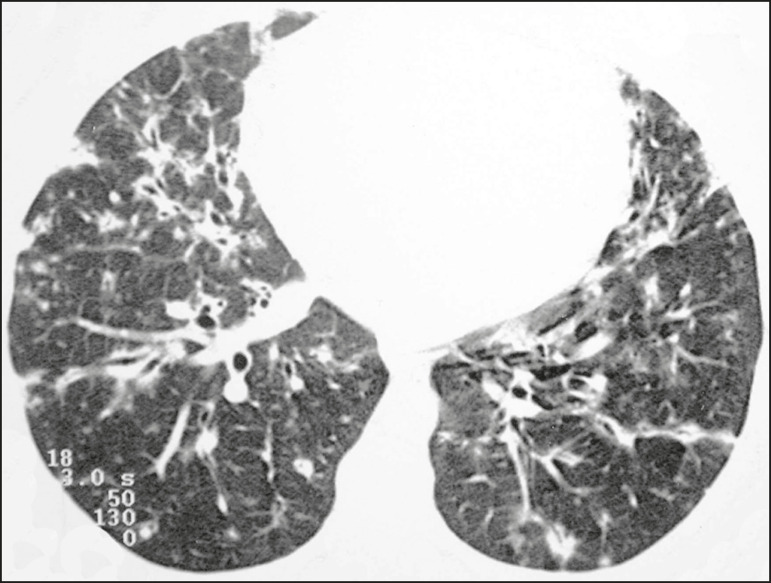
A 41-year-old woman with LIP related to Sjögren syndrome. Chest CT scan shows peribronchovascular thickening associated with multiple small nodules.
